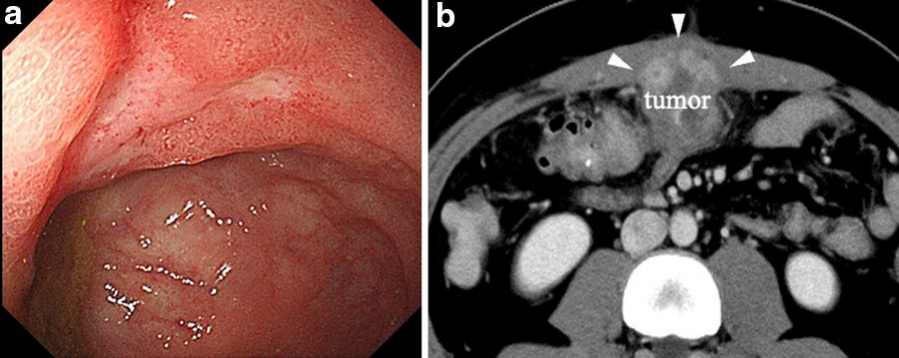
Colonoscopy and contrast-enhanced CT scan findings after emergent surgery. Colonoscopy reveals shrinkage of the tumor (a) and enhanced CT shows reduction of tumor invasion of the abdominal wall to about 5 cm (white arrowhead) (b)
